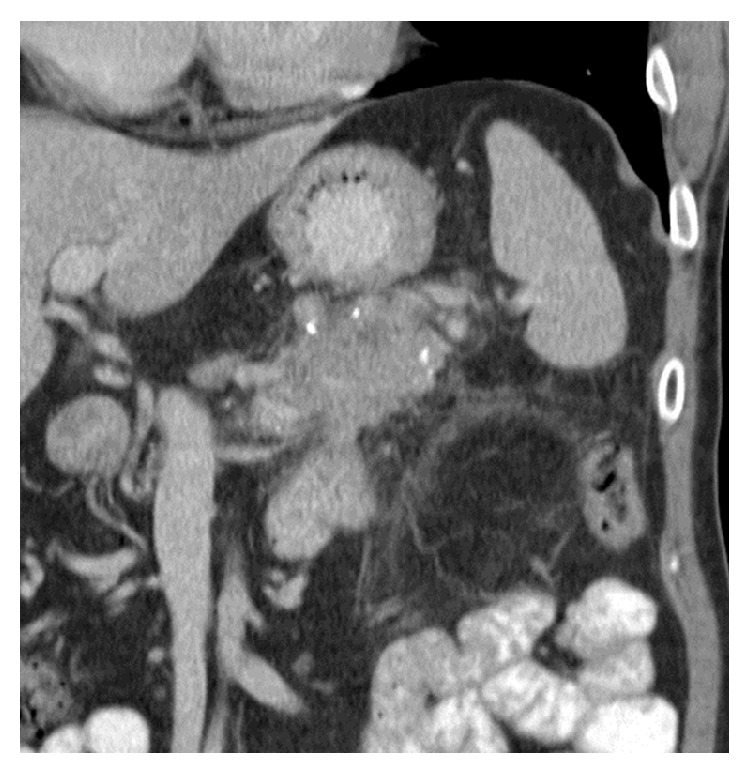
Coronal CT view: calcifying chronic pancreatitis with a heterogeneous mass in the pancreatic tail.
